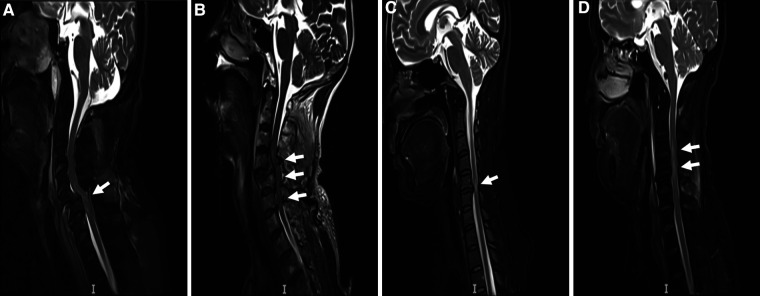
Two typical MRI scans of TCSCI patients. MRI scans of TCSCI patients with fracture/dislocation and disc herniation/bulging. (A) The C5 vertebral body slipped forward to the III° position; the spinal canal was narrowed and the cervical spinal cord was severely compressed. (B) The C4 vertebral body slipped forward to the II° position and a TCSCI is evident at the C4 -6 level. (C) The C5/6 intervertebral disc herniated backward and a TCSCI is apparent at the C5/6 level, especially C5. (D) The C3/4, C4/5, C5/6, and C6/7 intervertebral discs herniated posteriorly and a TCSCI is apparent at the C3/C4 level.
